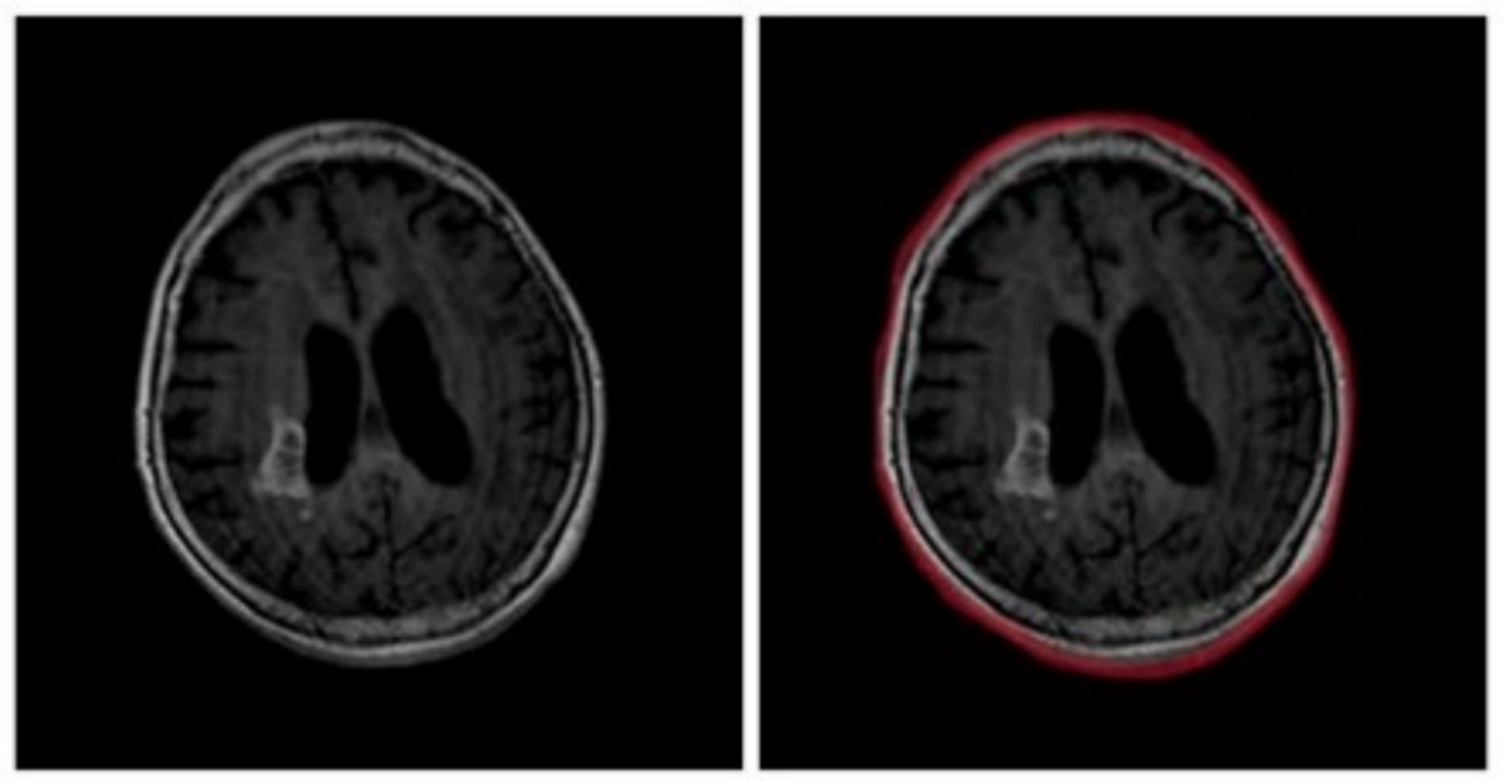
Tumor contour of MRI images.
